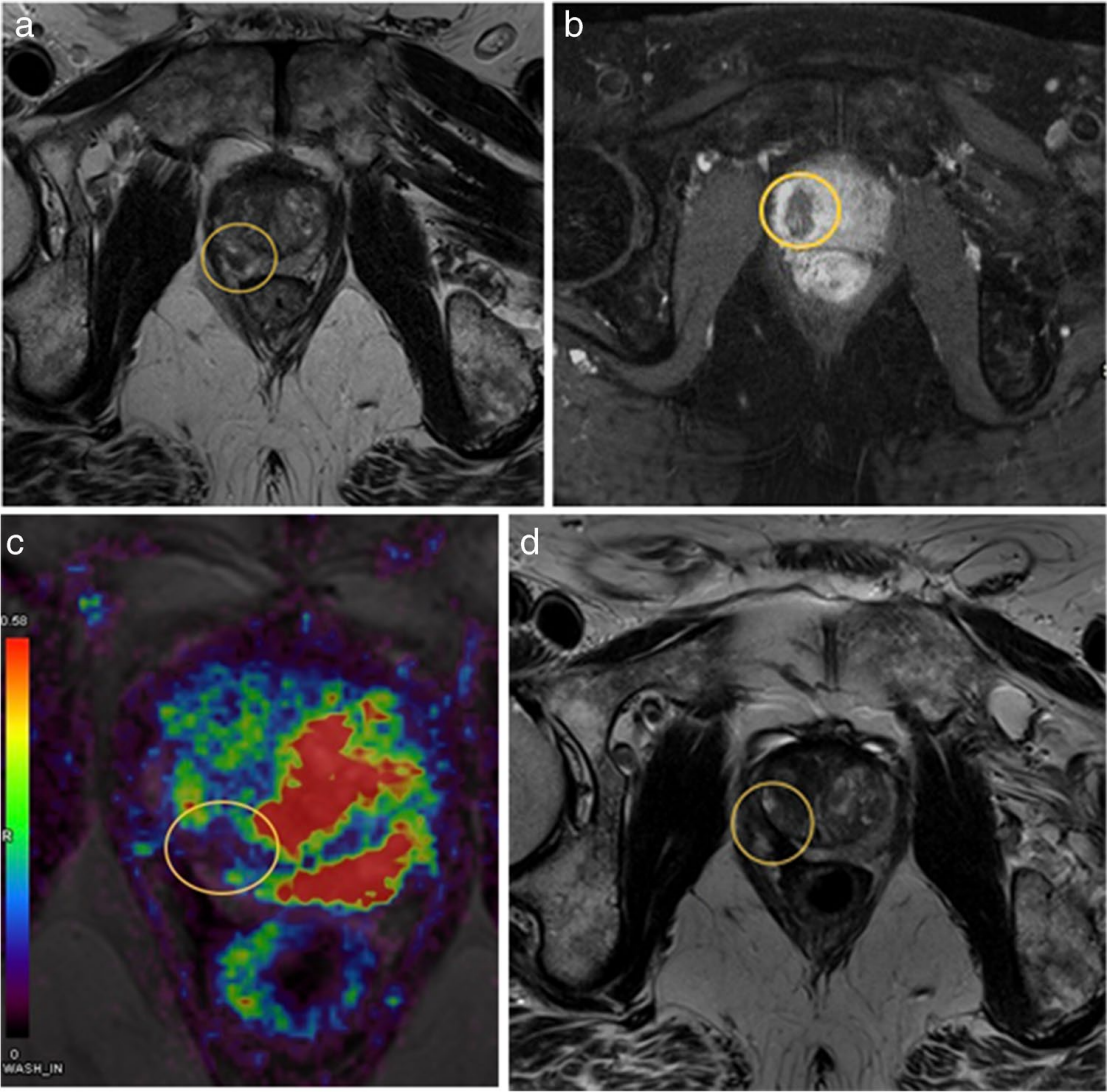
A 68-year-old male presenting with an elevated PSA level of 5 ng/mL was diagnosed with prostate cancer in the right peripheral zone in the midportion of his prostate, Gleason score of 3 + 4 = 7 (ISUP 2). a T2-weighted MR image of the lesion before treatment. b Contrast enhanced image directly at the end of the focal laser ablation procedure, showing lack of enhancement at the ablation bed. At 1-year follow-up after treatment, the patient’s PSA level dropped to 2.2 ng/mL and the following MRI images confirmed no evidence of residual or recurrent tumor at the treatment area: c Perfusion weighted color MR image, showing lack of perfusion in the treated area. d T2-weighted MR image, showing complete focal atrophy of the treated area
